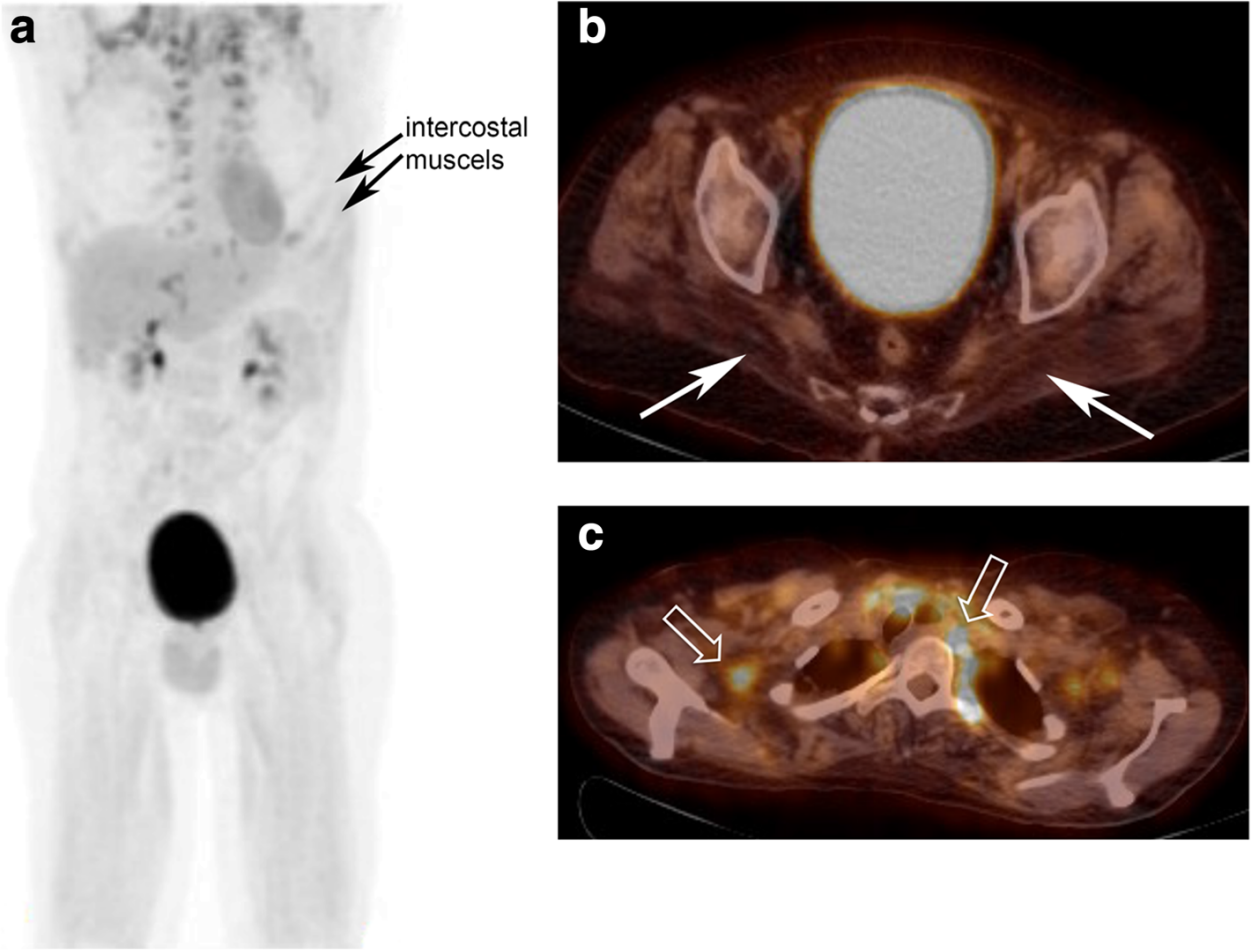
a Maximum intensity projection image showing the paravertebral brown fat as well as intercostal muscle. b Upper panel showing fused FDG PET/CT image with gluteus muscle atrophy and almost no FDG-uptake (white arrows). c Lower panel showing paravertebral and axillary brown fat (open arrows)
